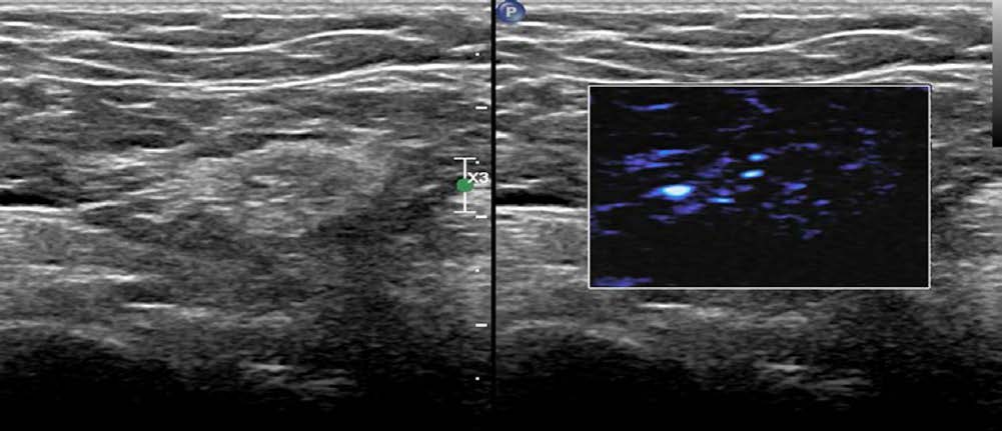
Ultrasonography, showing the axillary lymph nodes had normal hilums.
